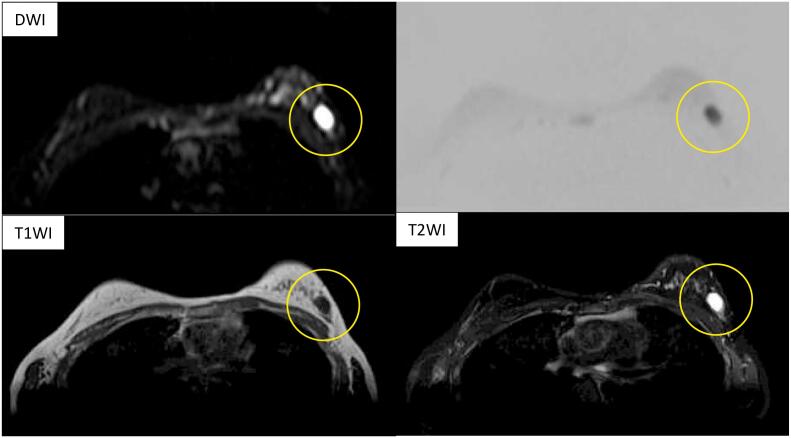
Magnetic resonance imaging reveals a relatively clear tumorous lesion with the high-intensity signal on T2WI and DWI, and the low-intensity signal on T1WI.
